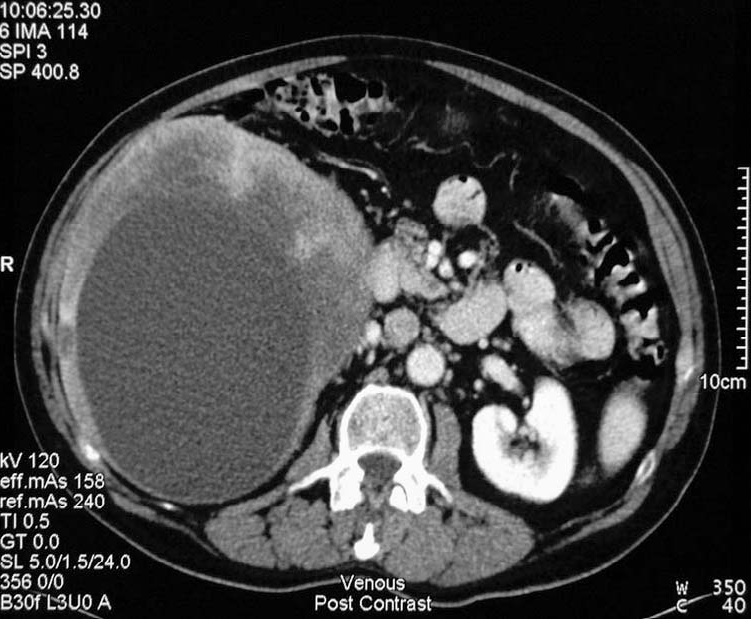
Portal venous phase image of an axial CT cut showing a large heterogeneous cystic mass within the right lobe of the liver centered on segment 5 and 6 and measuring 15 × 17 × 20 cm in maximum dimension. Further cuts show extension to segment 4A and segment 1 encroachment. No direct portal or peri-coeliac lymphadenopathy was identified.
